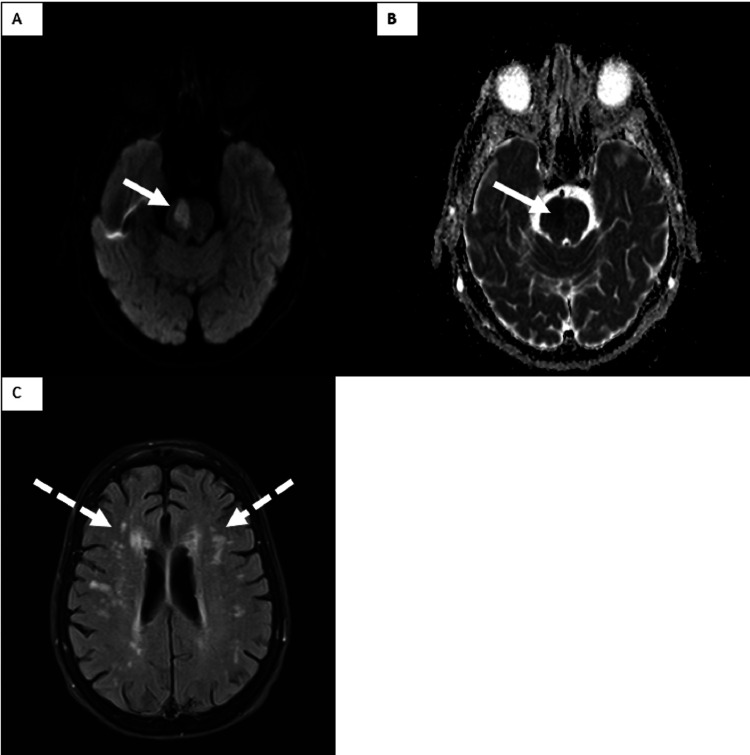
Axial MRI images (A,B) DWI and corresponding ADC showing an acute right hemi-pons lesion (solid arrow) (C) FLAIR sequence showing multiple T2/FLAIR hyperintensities in a supratentorial deep, subcortical, and periventricular white matter consistent with chronic microvascular ischemic changes (dashed arrows) DWI, diffusion-weighted image; ADC, apparent diffusion coefficient
